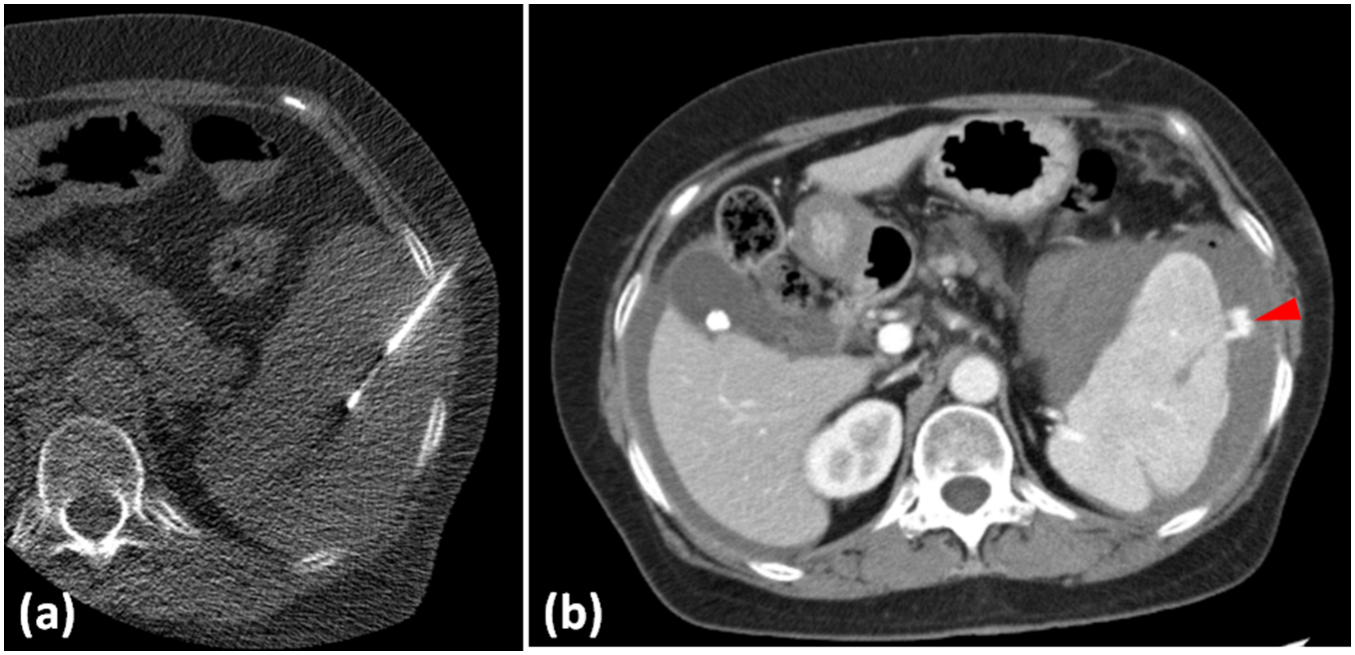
A 64-year-old woman with pancytopenia underwent splenic biopsy. (a) Splenic biopsy was performed under computed tomography (CT) guidance. (b) Immediately after biopsy, contrast-enhanced CT showed a peritoneal hematoma and extravasation around the spleen (arrowhead). Transcatheter arterial embolization was immediately performed.
